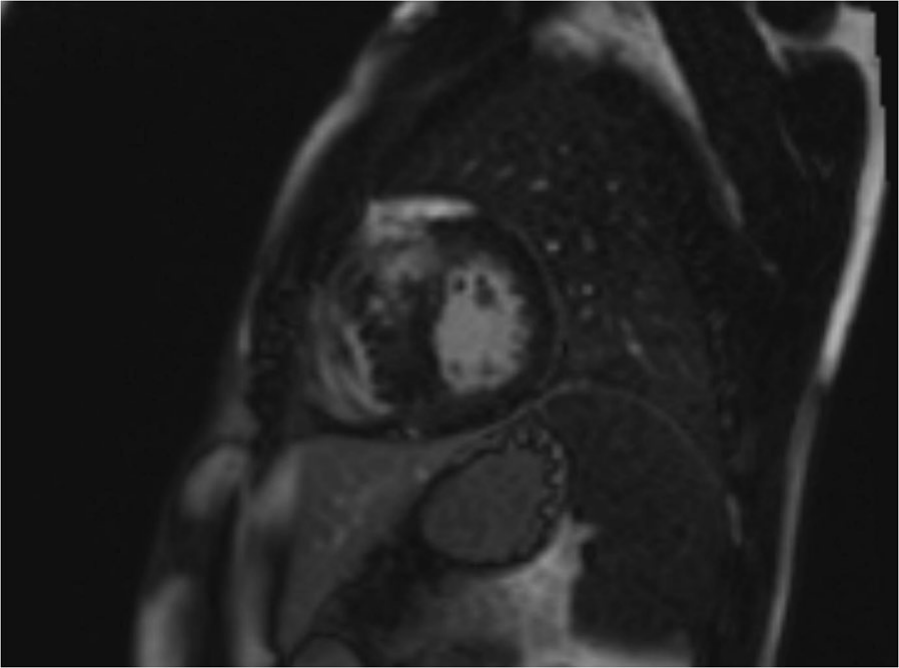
MRI short axis with delayed gadolinium enhancement
